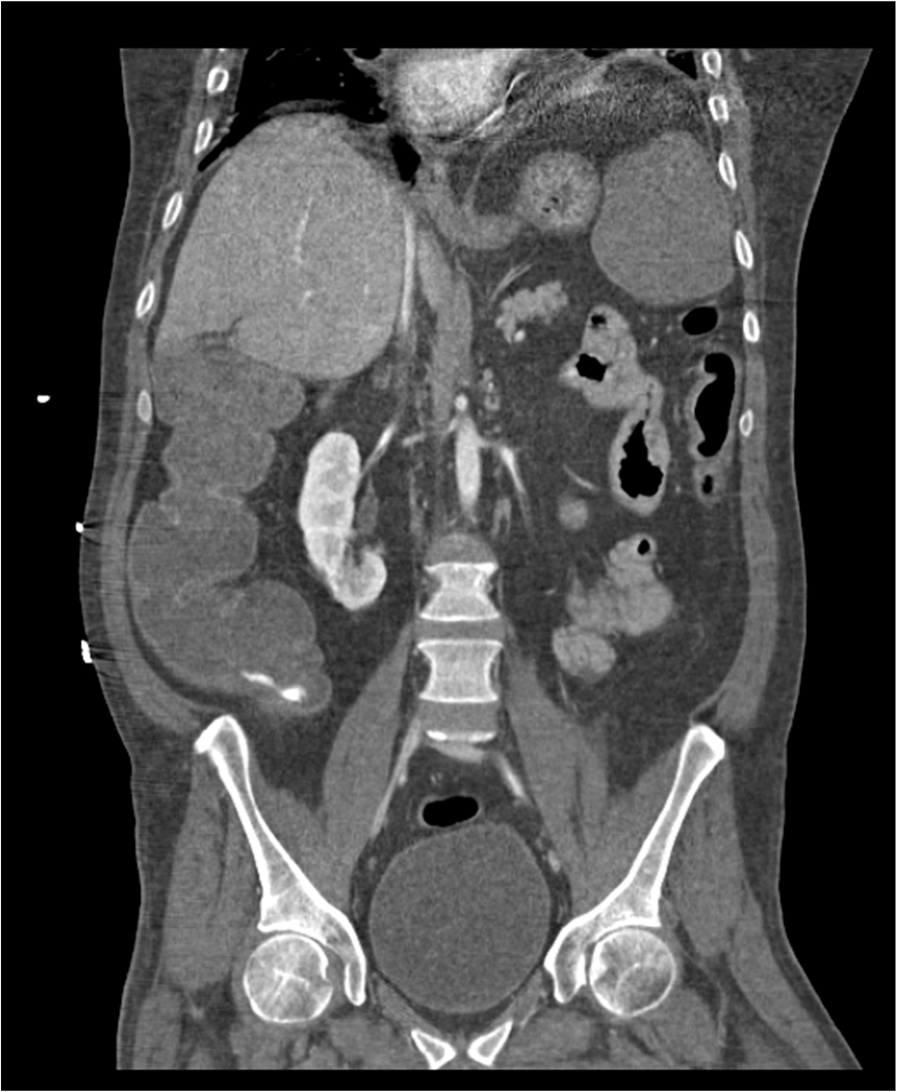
Coronal CT-angiography image shows active arterial bleeding in the cecum
